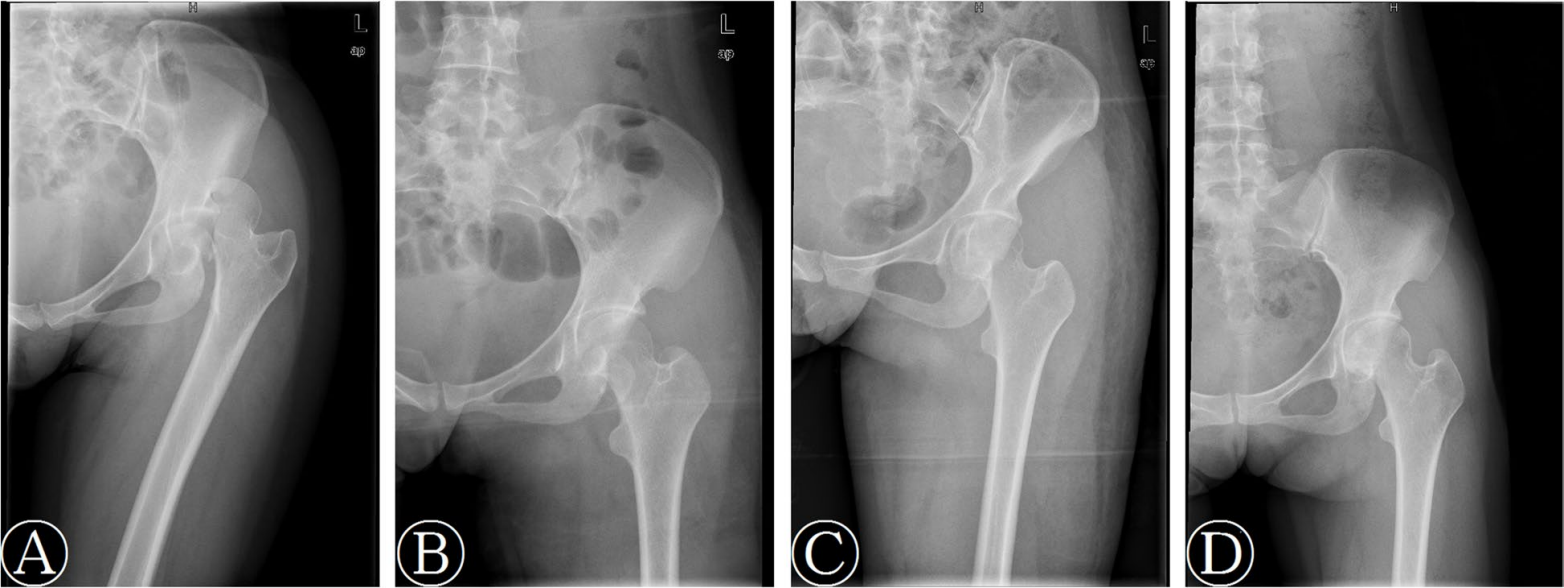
A 30-year-old woman with left Pipkin type II fracture. A Radiograph before reduction. B Radiograph after the reduction of posterior hip dislocation. C-D Post-operative and final follow-up radiographs
